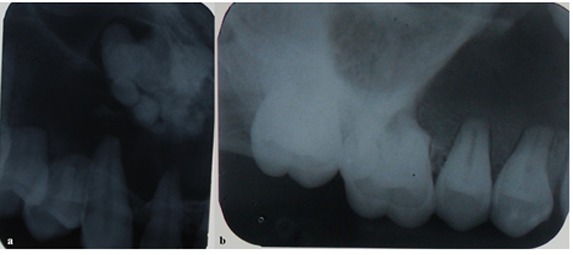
a: Intraoral radiograph showing radiopacity apical to tooth #22, #23  b: Intraoral radiograph showing root resorption with tooth #24, #25, mesiobuccal root of #26
